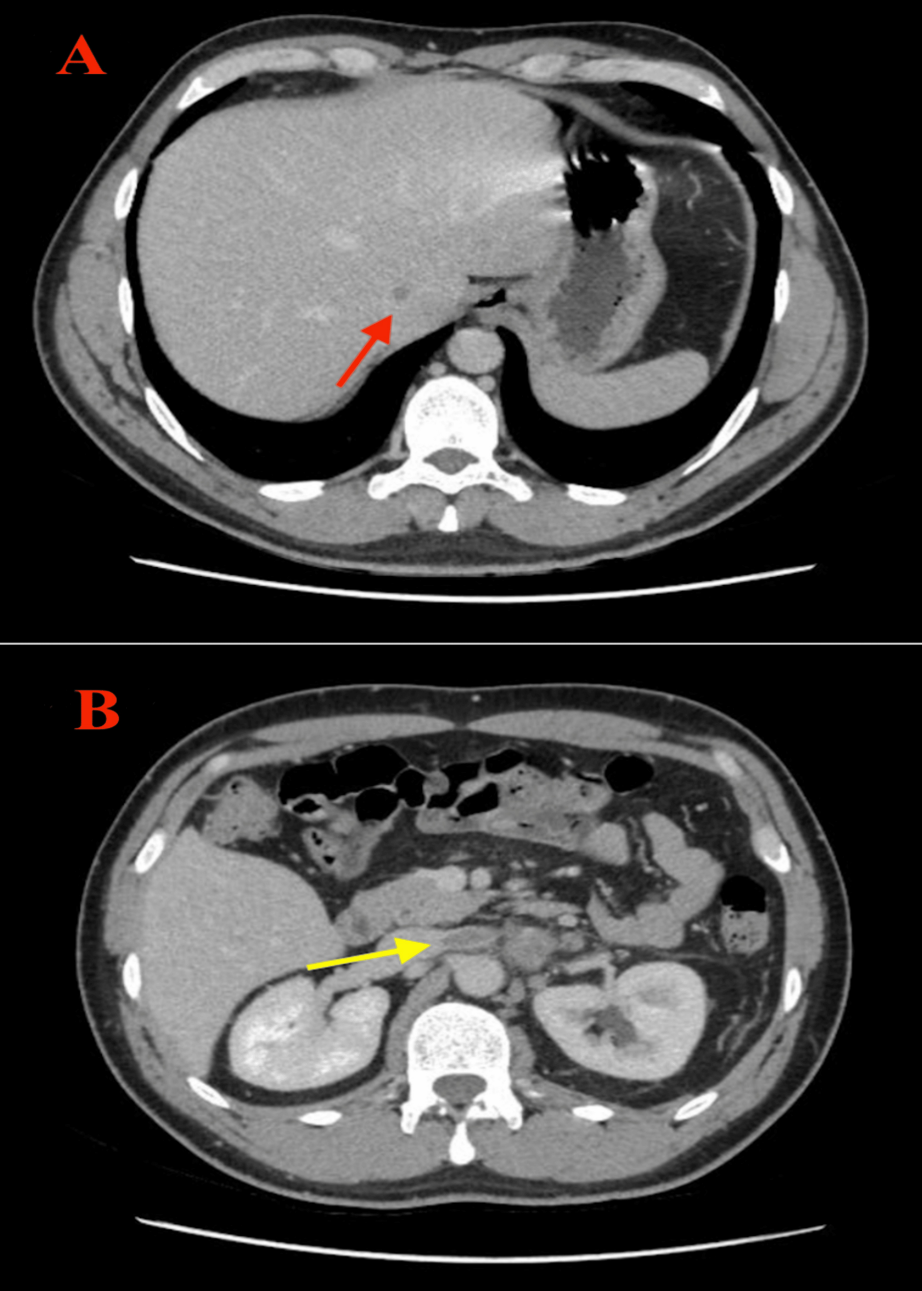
CT scan of abdomen A: The defect from the beginning of the vena cava (red arrow). B: The extension to the left renal vein (yellow arrow).
